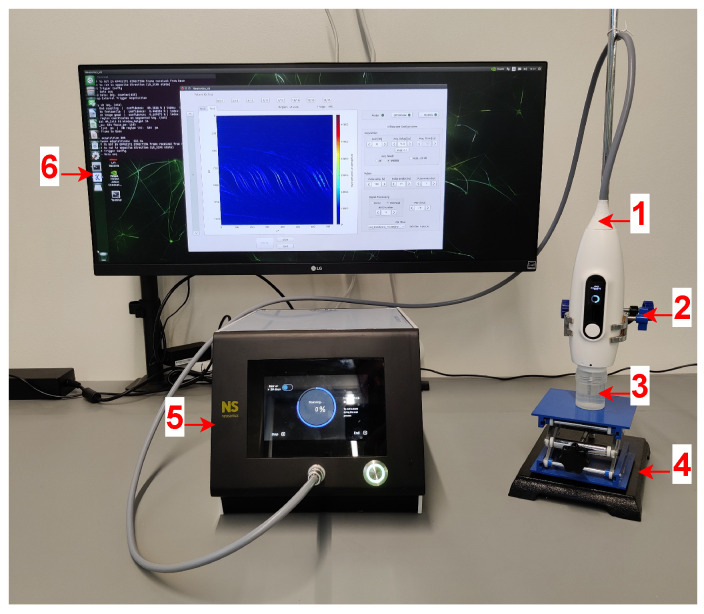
Experimental setup composed of (1) ultrasound probe, (2) bracket, (3) sample container, (4) elevating platform, (5) base module, and (6) external monitor.
